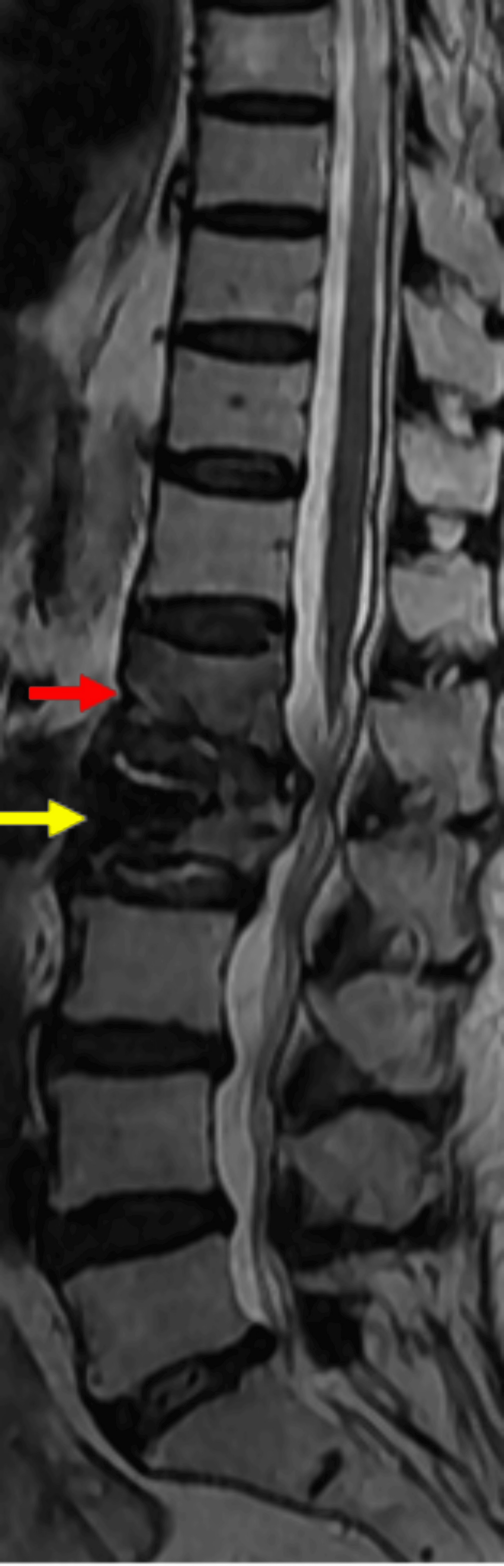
Follow-up sagittal T2-weighted magnetic resonance imaging of the thoracolumbar spine showing post-vertebroplasty status at L2 (yellow arrow) with a new adjacent-level compression fracture involving the L1 vertebral body (red arrow).
